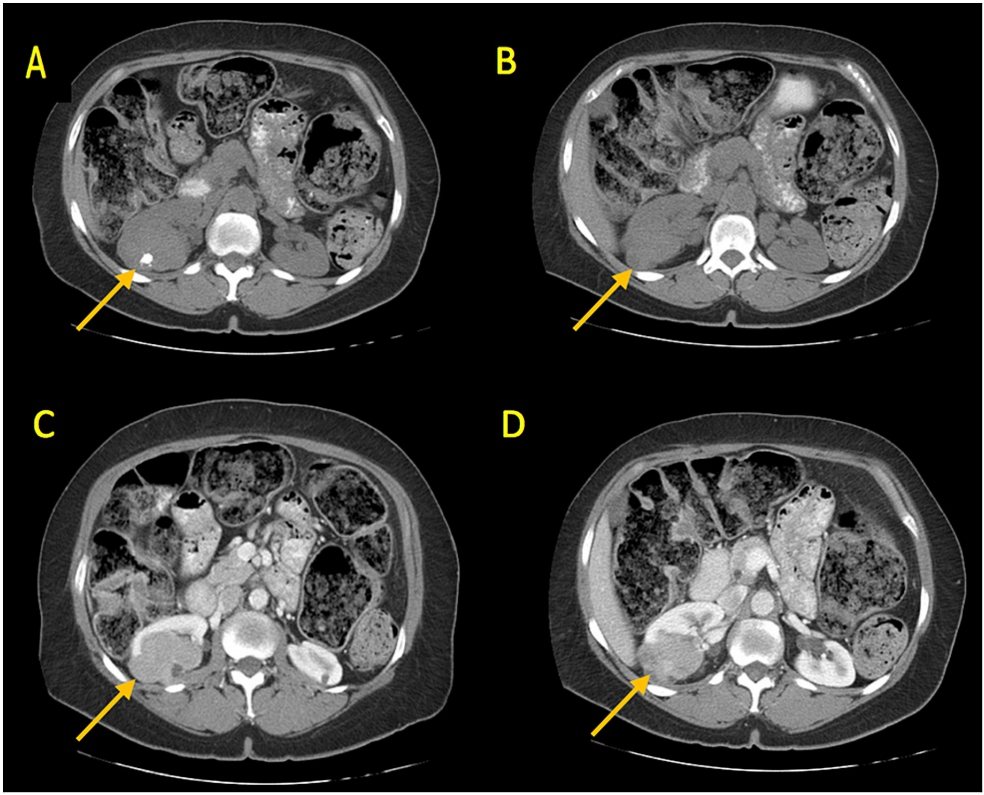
CT scan of abdomen and pelvis. Non-contrast exam demonstrating (A, B) and contrast (C, D) 7-cm prominently solid but enhancing dorsal right mid renal lesion with no evidence of osseous, adrenal, or hepatic metastasis or renal venous invasion or retroperitoneal lymphadenopathy as demonstrated by the arrow.
